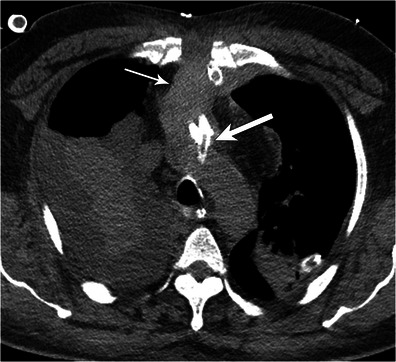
Haematoma. Axial CT in a 44-year-old male on mediastinal ECMO for heart failure after myocardial infarction shows mediastinal haematoma (thin arrow) tracking along the arterial cannula (thick arrow) up to the aortic arch
